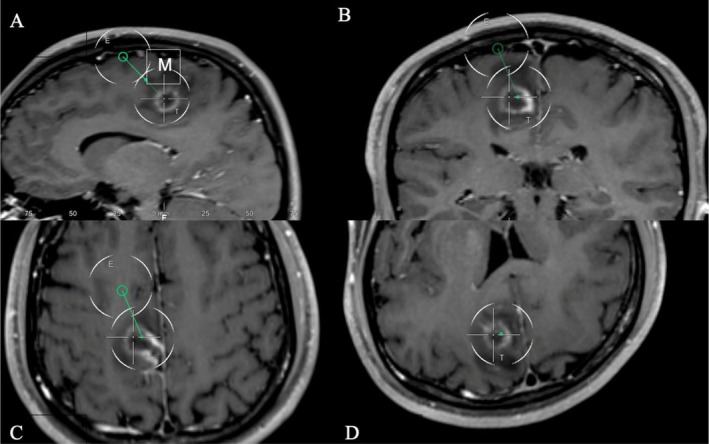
Preoperative planning of the surgical trajectory for aspiration of the abscess. The green circle represents the entry point of the aspiration needle, and the intersecting lines represent the target for aspiration. The letter “M” denotes the motor cortex. The trajectory is demonstrated in the (A) sagittal, (B) coronal, (C) axial, and (D) perpendicular axis on a T1‐weighted fat‐saturated, post‐gadolinium, contrast‐enhanced MRI.
